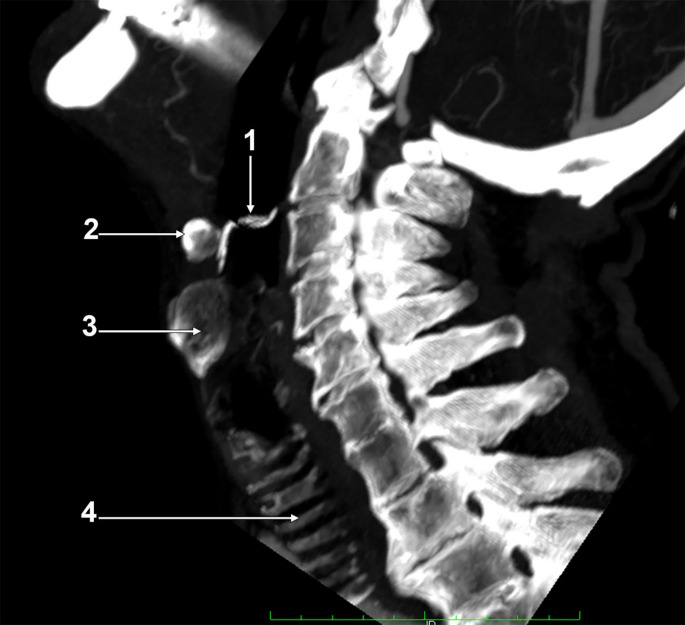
Sagittal CTA slice showing a mineralised epiglottis. (1) epiglottis; (2) hyoid body; (3) thyroid cartilage; (4) trachea
